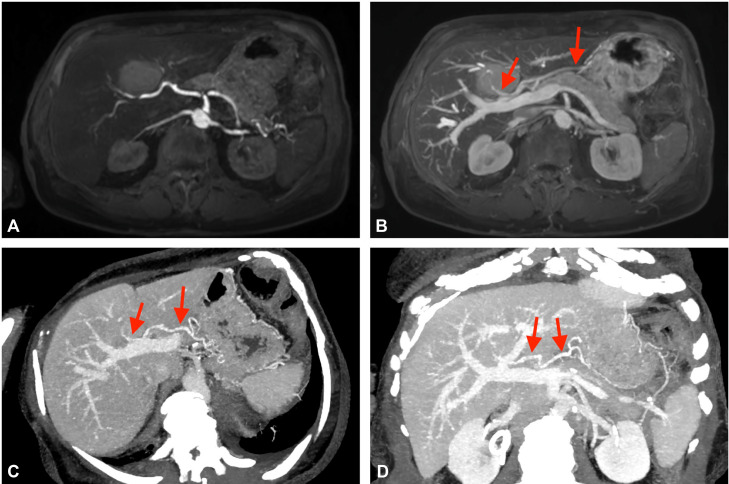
Dixon sequence in (A) arterial and (B) portal venous phase showing accessory perfusion of posterior segment 4 by an aberrant right gastric vein (*arrows*). (C) + (D) Different multiplanar reformations (MPRs) of the aberrant right gastric vein in a contrast-enhanced CT in portal venous phase (*arrows*). The vein originates from the lesser curvature of the stomach and directly supplies segment 4 of the liver. Note the different contrast enhancement between the aberrant venous vessel and the arterial vessels (ie, the celiac trunk and the common hepatic artery).
